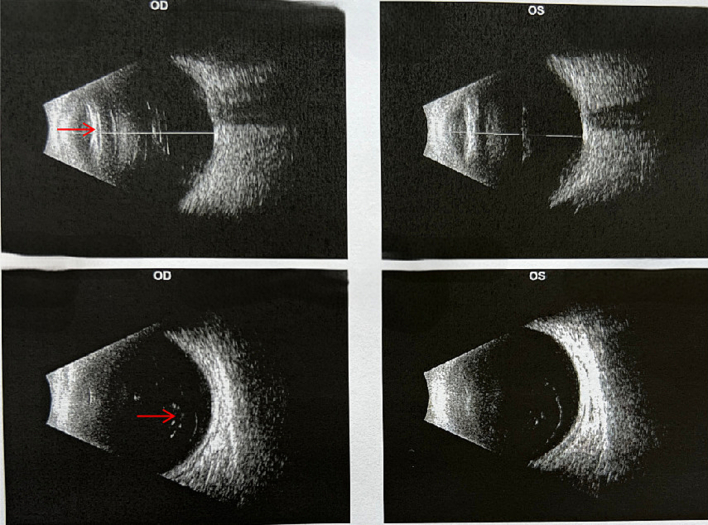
Preoperative ocular ultrasound examination. The crystalline lens of the right eye was cloudy. The vitreous humor of both eyes was cloudy, and no other significant abnormalities were observed.
